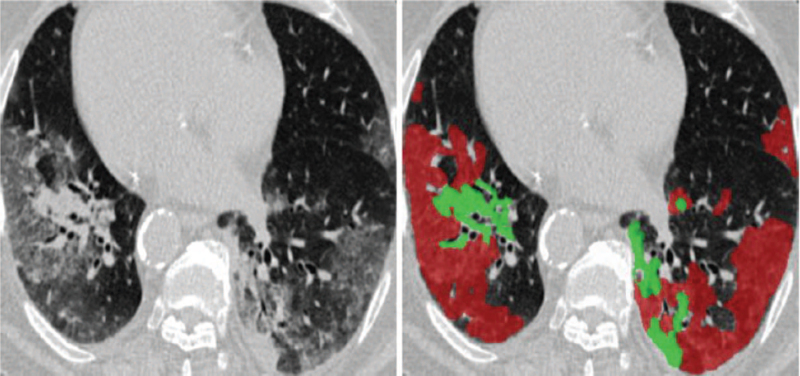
COVID 19-infected region exemplary CT axial slices, wherein the red and green mask and the mask represent consolidation GGO.^[62]^
